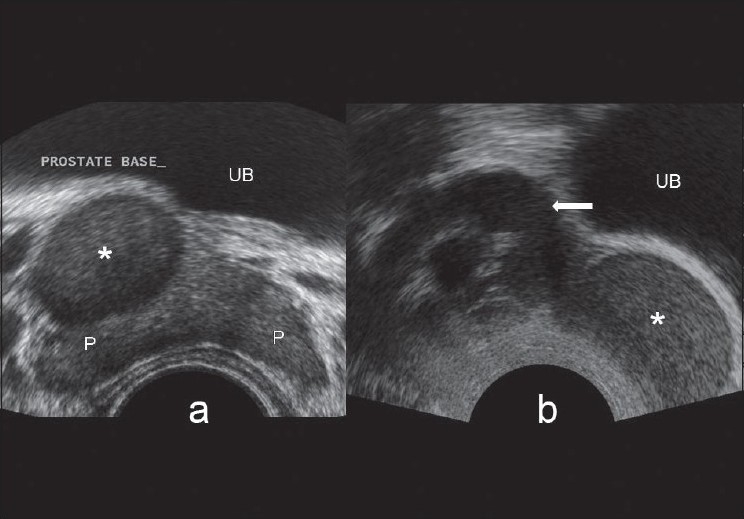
(a) Trans-rectal ultrasound transverse image showing a large cyst (*) with internal echoes in the region of right seminal vesicle. (b) Trans-rectal ultrasound parasagittal image showing a large cyst (*) with internal echoes in the region of right seminal vesicle. Gross dilatation of the proximal part of right seminal vesicle is also seen (arrow). P, prostate; UB, urinary bladder
